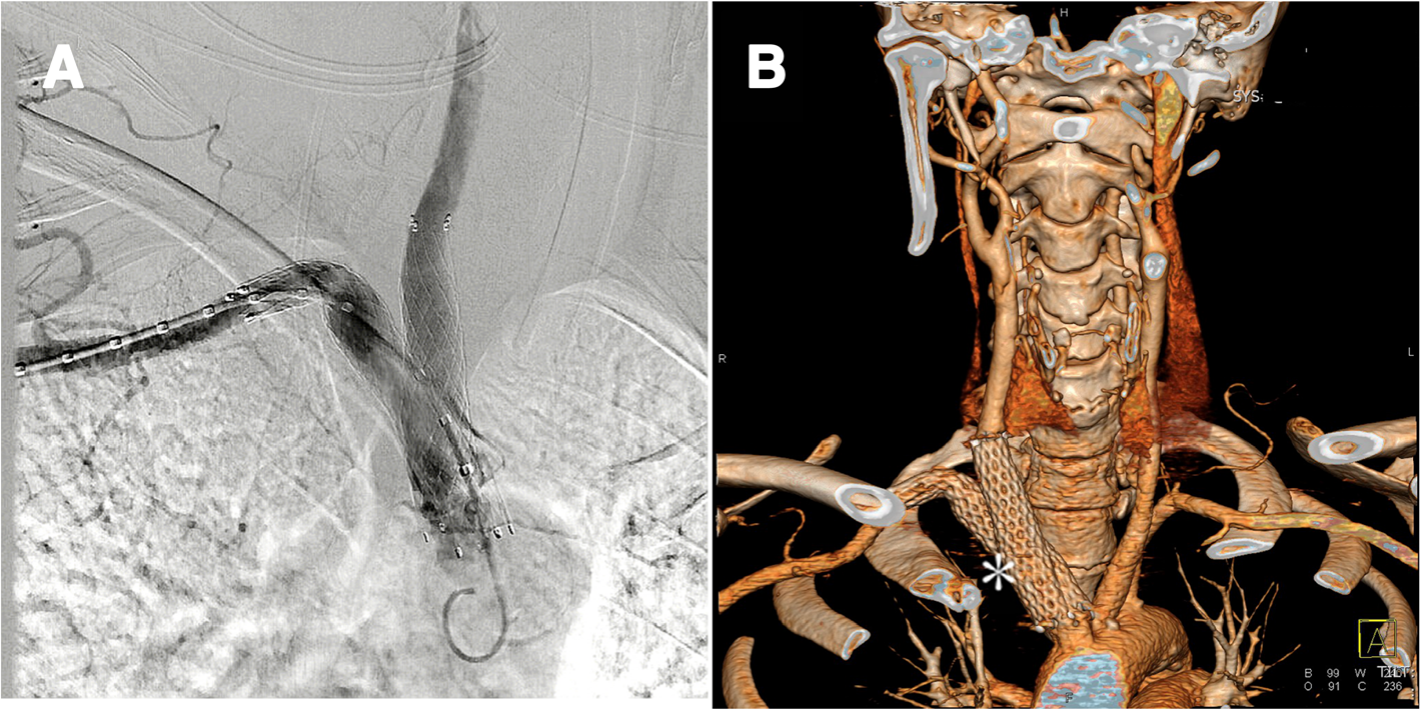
a After deployment of two stent grafts, digital subtraction angiography shows that no contrast goes into the pseudoaneurysm anymore, and both carotid artery and subclavian artery are patent. b Two-week follow-up computed tomography angiography of the patient, *white star* (*) shows the two “kissing stent grafts” are in the right position and with no contrast leak. Furthermore, both carotid artery and subclavian artery are patent
